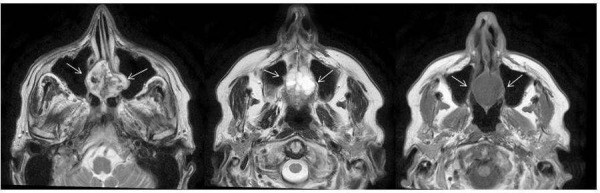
Magnetic resonance imaging (MRI) scan at diagnosis. The arrowheads indicate the lesion.
